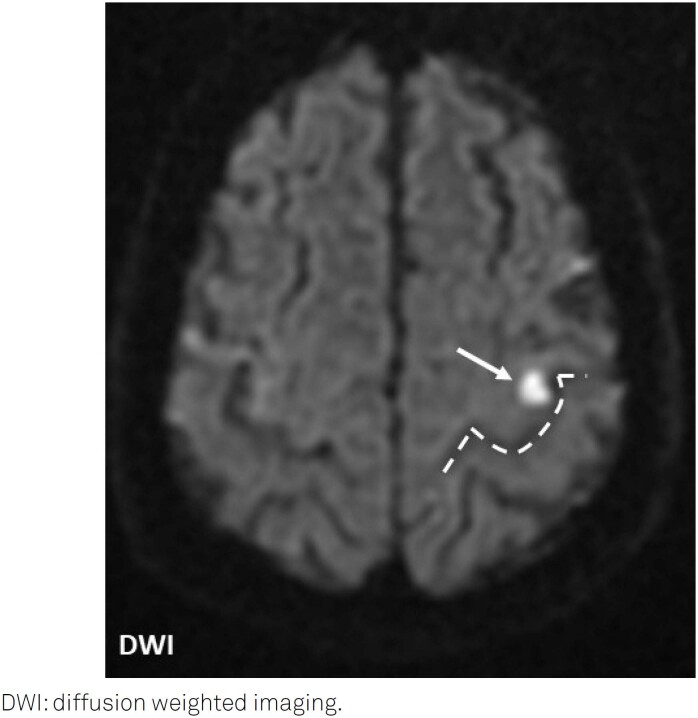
Brain magnetic resonance imaging (axial section) displaying an acute infarction (white arrow) in the hand knob territory, presenting as an inverted omega (dashed line).
